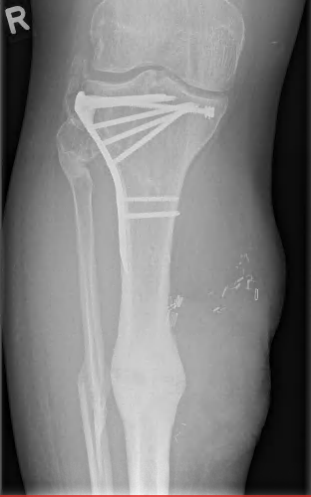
Posteropative X-rays after 1 year
